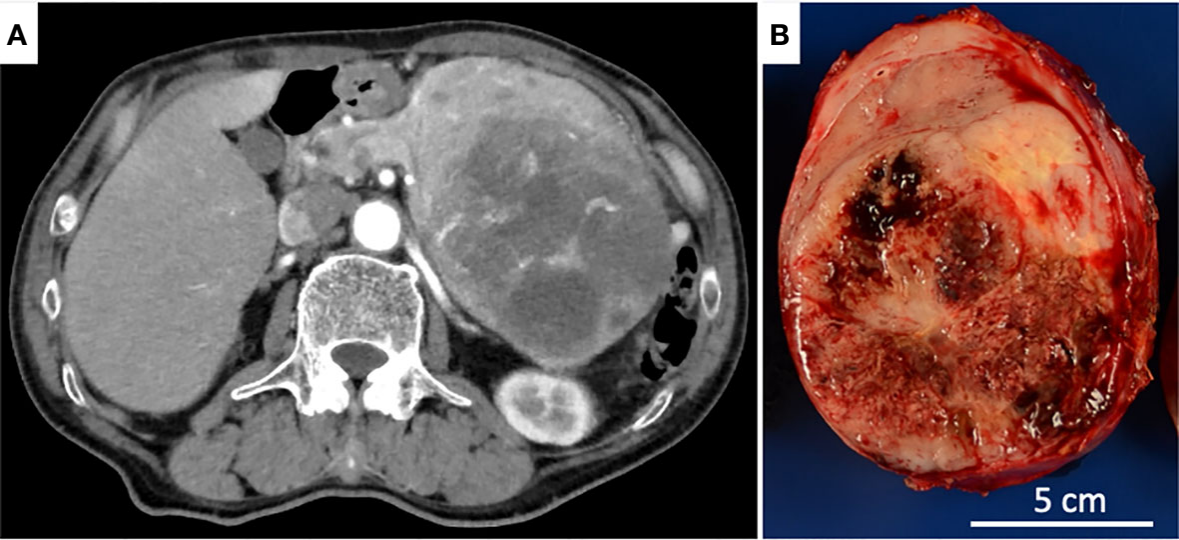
(A) Axial CT revealed an expansile, solid tumor largely degenerated with necrosis in the dorsal part. (B) The cut surface of the pancreatic tail tumor showed a well-circumscribed, lobulated, solid tumor with a large hemorrhagic, necrotic area.
